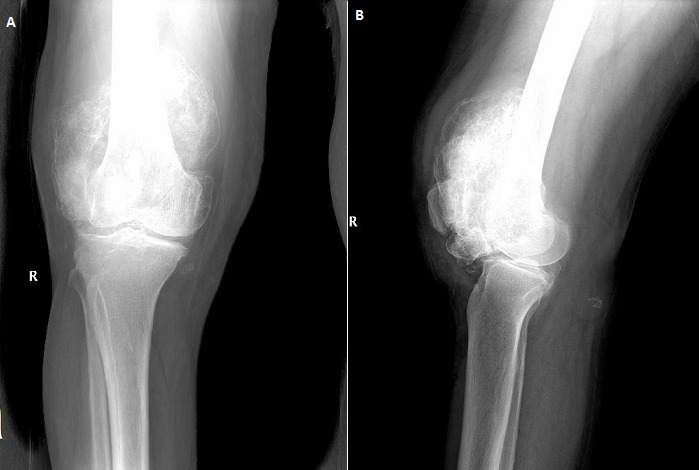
X-ray of right knee joint showing the radiodence body. Anteroposterior (a) and lateral (b) X-ray of the knee
